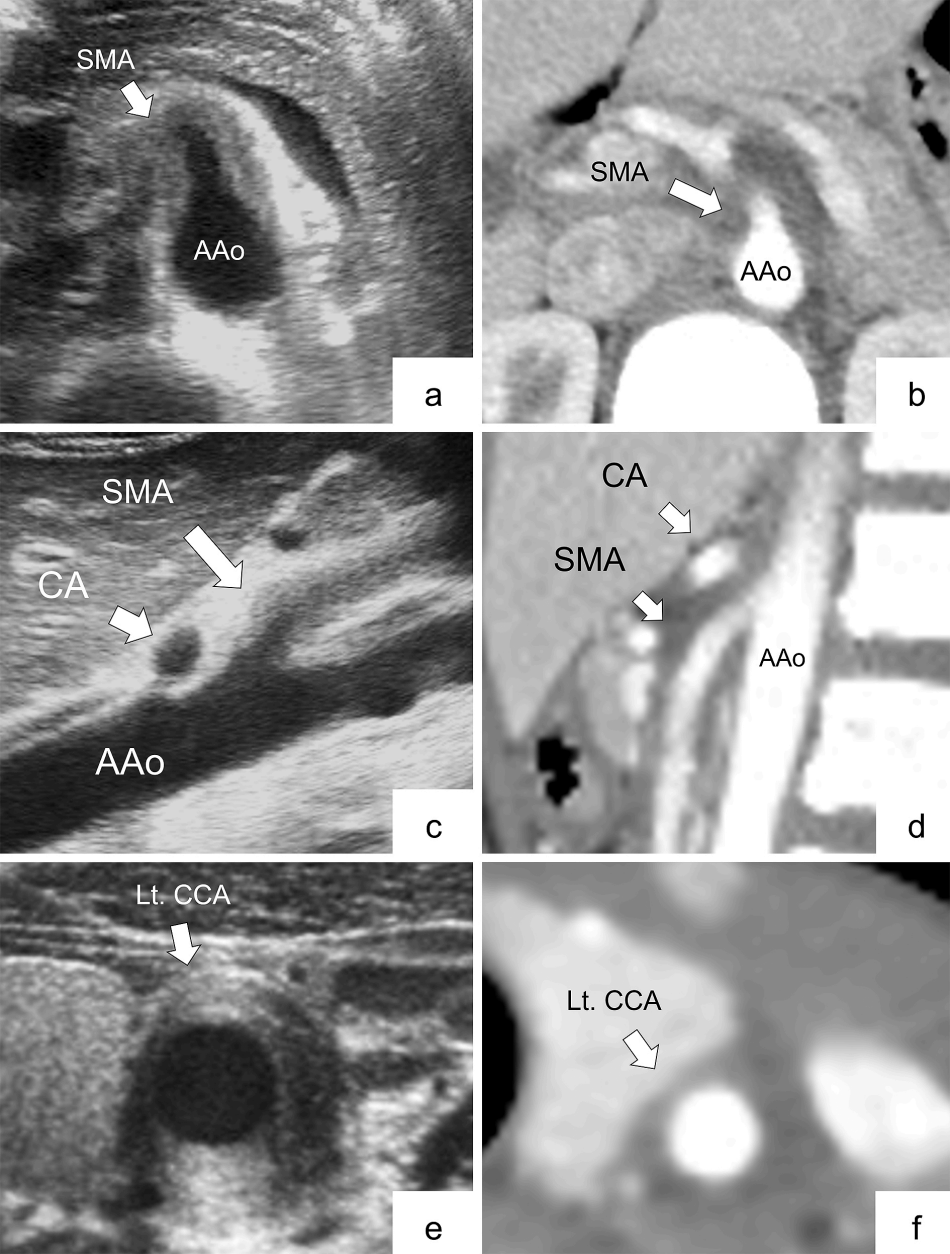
Representative images of arterial involvement comparing ultrasonography with contrast-enhanced computed tomography (CECT) in Patient No. 3 (panels a, b, c, d, e, and f). a: Transverse view shows wall thickening at the level of the trunk of the superior mesenteric artery (SMA) in the ultrasonogram (arrow). b: CECT at the same level of the abdominal ultrasonogram demonstrates wall thickening of the SMA (arrow). c: Longitudinal view shows arterial stenosis at the level of the trunk of the celiac artery (CA), and wall thickening of the SMA in the ultrasonogram (arrow). d: CECT at the same level of the abdominal ultrasonogram recognizes both findings of the CA and SMA (arrow). e: Wall thickening of the left common carotid artery (CCA) is shown in the transverse view in the ultrasonogram (arrow). f: Wall thickening of the left CCA is revealed by CECT at the same level of the neck ultrasonogram (arrow). AAo, abdominal aorta; CA, celiac artery; CCA, common carotid artery; Lt., left; SMA, superior mesenteric artery.
